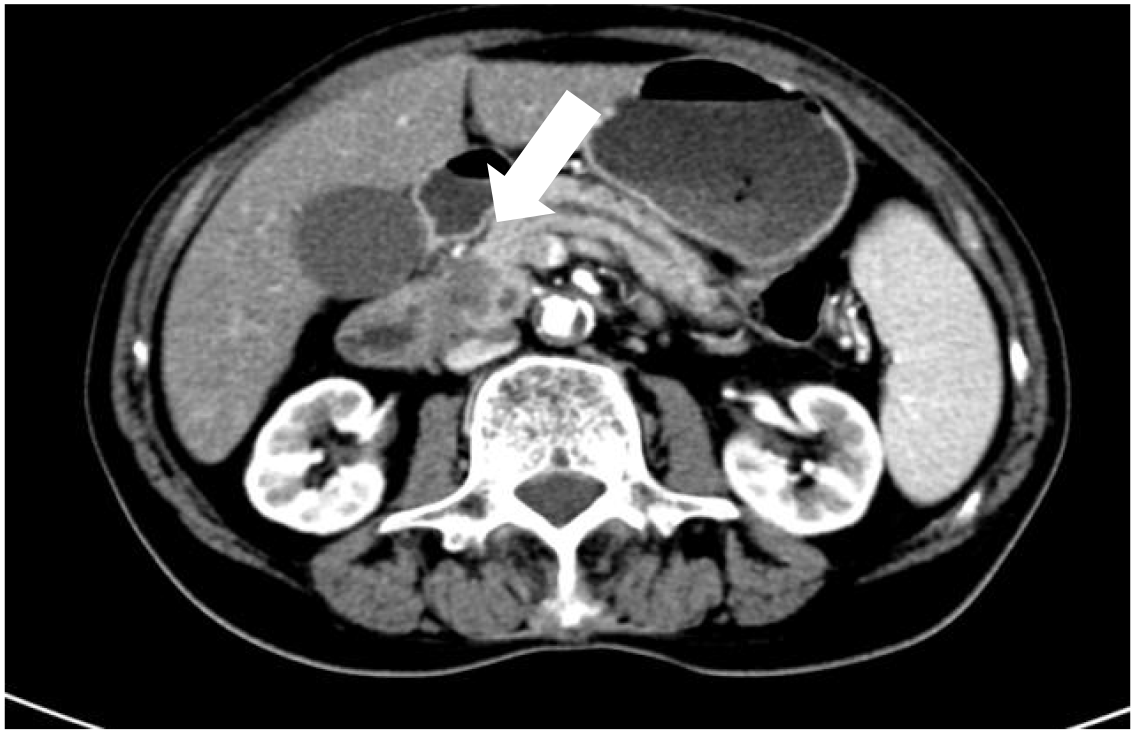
Contrast-enhanced CT shows an occupying lesion in the pancreatic head invading the duodenum.
